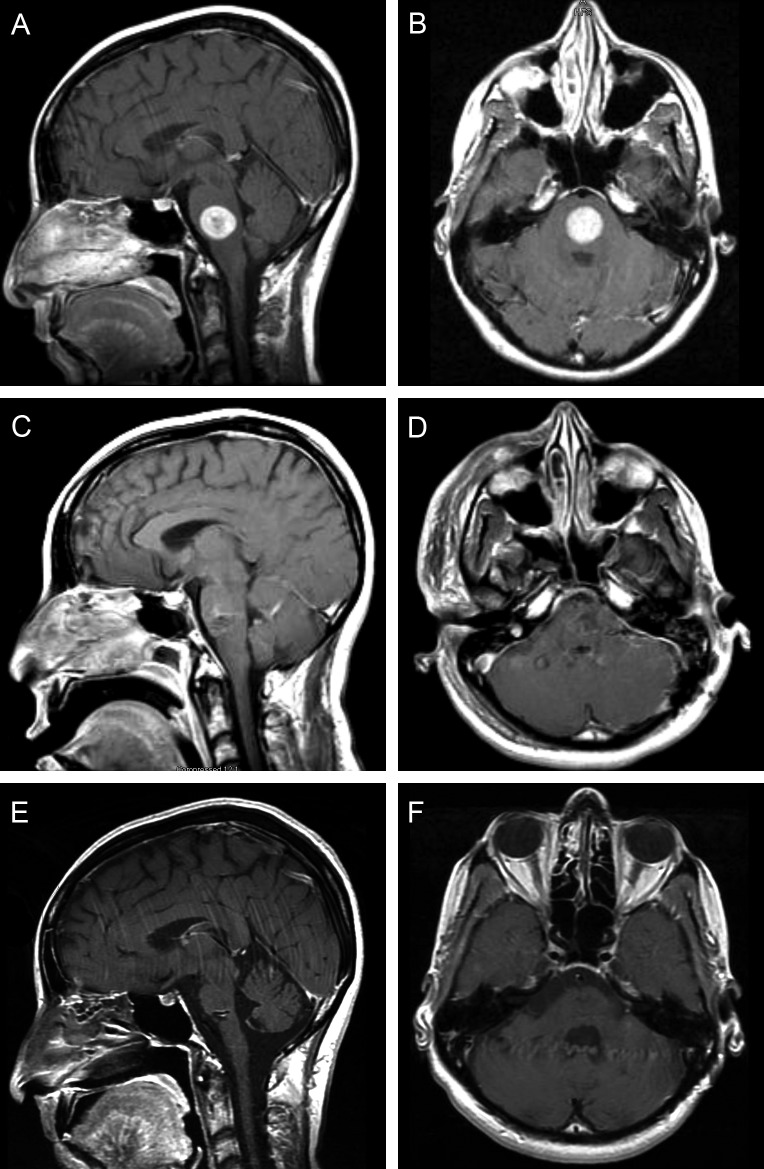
MRI study of the patient's metastatic lesion Initial sagittal (A) and axial (B) magnetic resonance imaging (MRI) scans with contrast of the head demonstrating a well-circumscribed enhancing lesion with surrounding edema in the pons. Postoperative MRI (C-D) with contrast demonstrating a gross total resection of the solitary lesion with no evidence of residual disease. MRI with contrast at 10 years (E-F) after the original craniotomy showed no signs of recurrent disease. *Used with permission from Barrow Neurological Institute, Phoenix, Arizona.*
